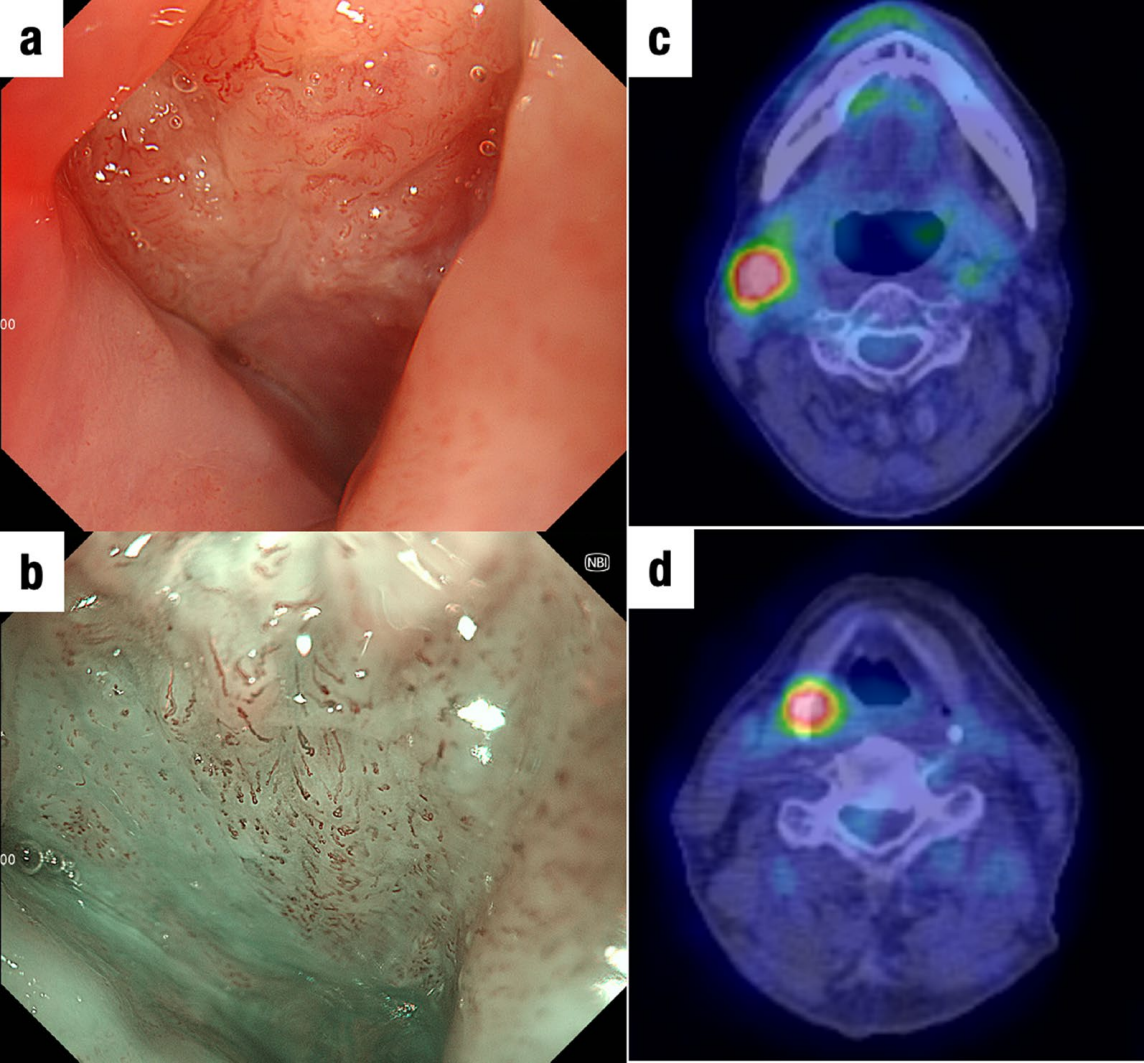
Preoperative examination. a Upper gastrointestinal endoscopy showing a ridge on the right piriform fossa. b Image-enhanced endoscopy showing an irregular granular surface and microvessel pattern in the right piriform fossa. c Fluorodeoxyglucose (FDG) positron emission tomography (PET) showing high FDG uptake in the right cervical lymph node. d FDG-PET showing high FDG uptake in the right piriform fossa
